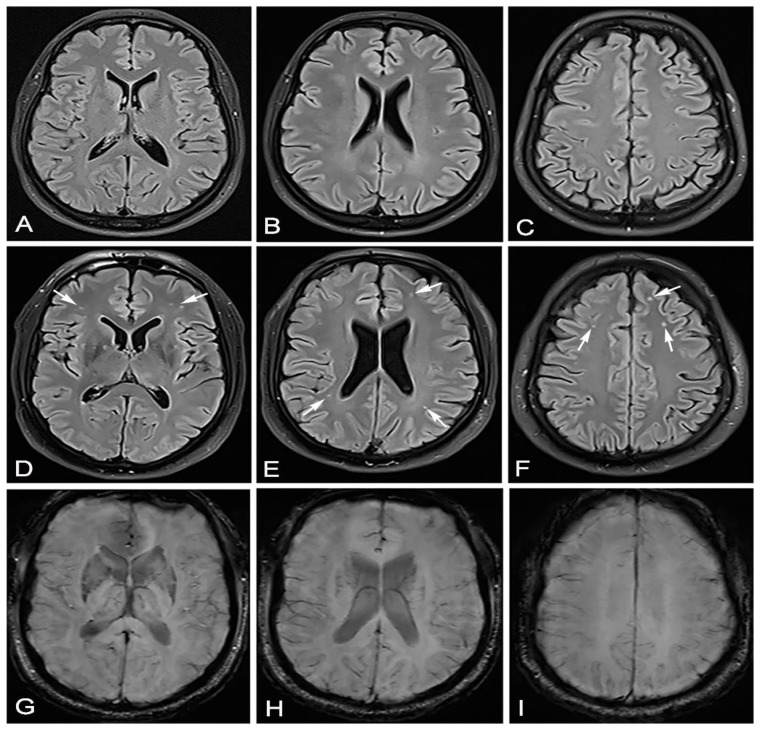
In June 2020, this male patient—as in Figure 1—had a completely normal brain MRI after vertigo (A–C). In March 2021, 53 days after recovering from moderate SARS-CoV-2 infection, the same patient came for a brain MRI due to headache, vertigo and taste and smell disorder. Brain MRI showed hyperintensive lesions on the FLAIR sequence located mostly bilaterally frontally subcortically and bilaterally periventricularly (D–F) (white arrows). The same transverse cross sections of the FLAIR sequence as on (A–C), without signs of microhemorrhage on the SWI sequence (G–I).
